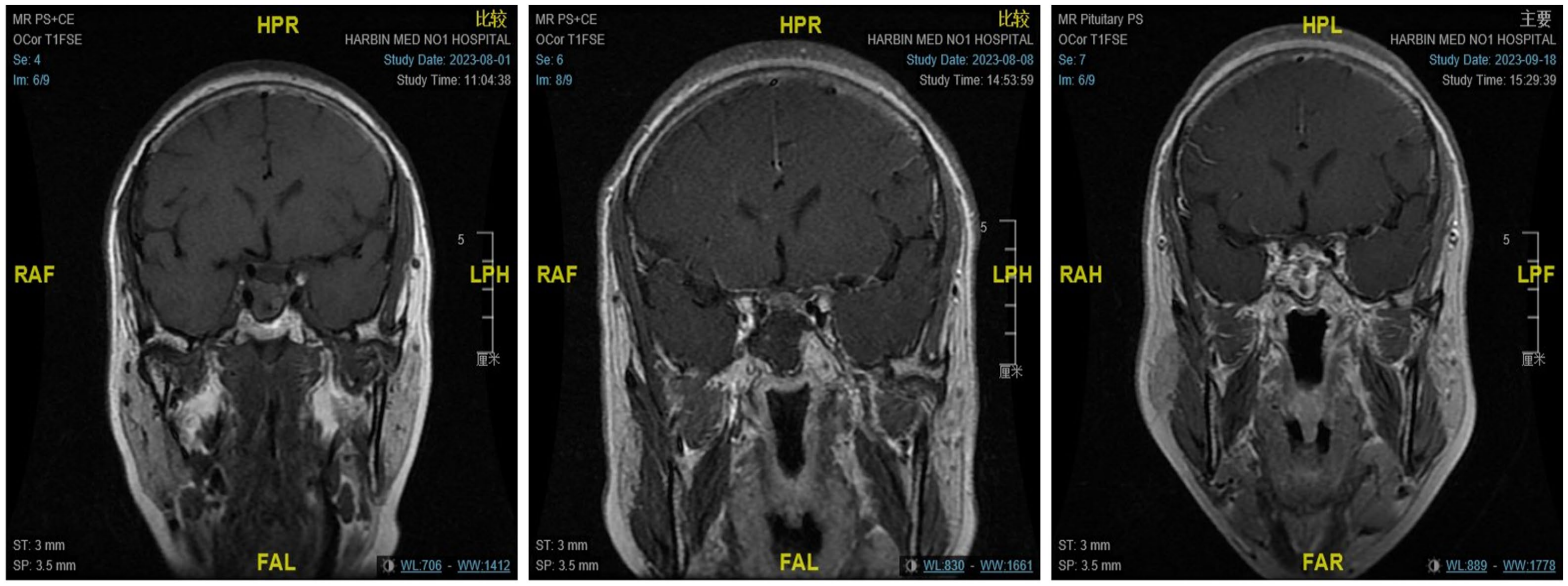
From left to right are the MRI scans of the patients before surgery, on the day after surgery, and 3 months after surgery, respectively.
